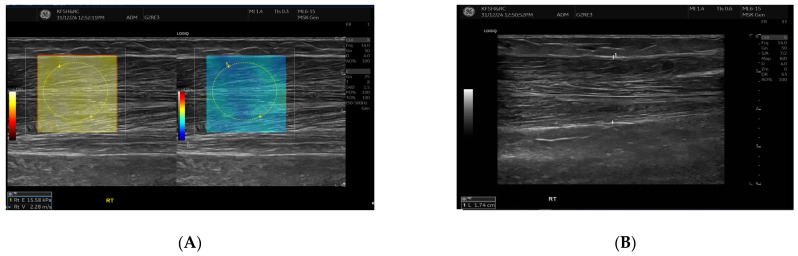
This figure shows the Ultrasound assessment of stiffness near the arteriovenous fistula site (A) and brachioradialis muscle thickness (B). Shear wave elastography images (A) obtained at the fistula side demonstrate quantitative stiffness mapping within a defined region of interest, with stiffness expressed in velocity in metres per second (m/s). B-mode image (B) showing brachioradialis muscle thickness measurement at the contralateral (right) arm.
